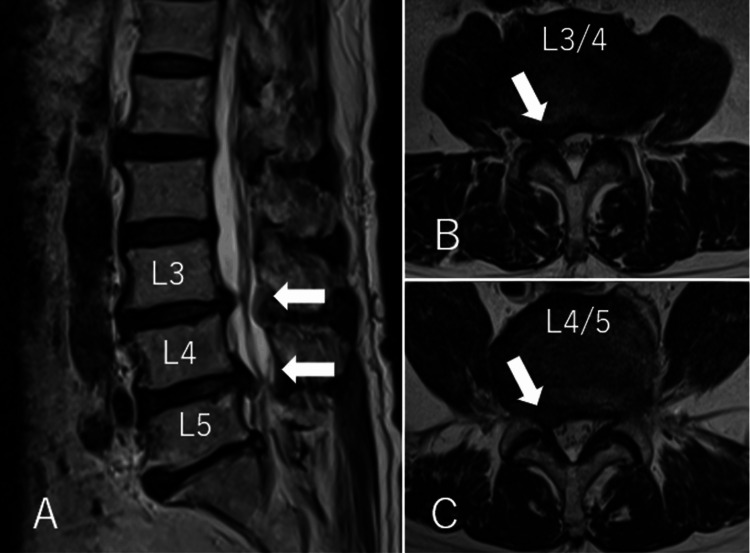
Preoperative MRI (A) Sagittal. (B, C) Axial. T2-weighted MRI performed preoperatively showing lumbar disc herniation (white arrow).
